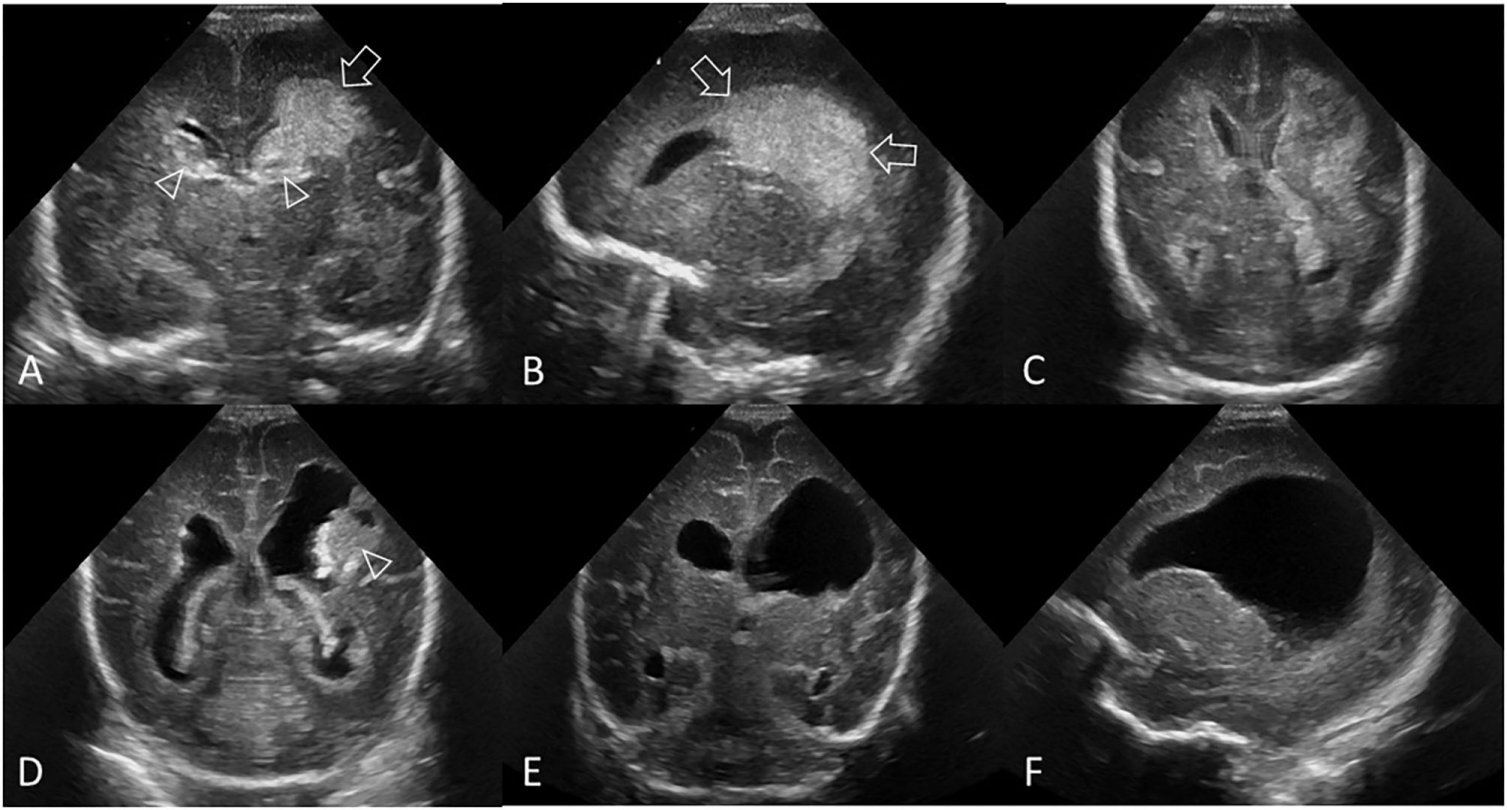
Grade III GMH-IVH + PVHI. Ultrasound scan in a preterm infant (GA 24 weeks) with bilateral GMH-IVH and left-sided PVHI. (A) Coronal scan 2 days after birth showing a left-sided grade III GMH-IVH (arrowhead, AHW = 7 mm, measurement not shown) and large echodensity in the left frontoparietal white matter (arrow). Smaller right-sided grade II GMH-IVH (arrowhead, AHW = 4 mm, measurement not shown). (B) Left parasagittal scan showing the periventricular echodensity (arrows) extending from the posterior frontal white matter to the parietal white matter. (C) Follow-up coronal scan 2 weeks after birth shows the area of PVHI beginning to develop central echolucency, indicating early cystic degeneration. (D) Follow-up coronal scan 7 weeks after birth shows further cystic degeneration following the PVHI with echogenic clot debris, dilated lateral ventricles, and echogenic ependymal lining. (E,F) Follow-up coronal and left parasagittal scans at 12 weeks show the large porencephalic cyst communicating with the lateral ventricle. Internal clot debris has cleared and ependyma is no longer echogenic. The persisting ventriculomegaly is now partially passive (i.e., ex-vacuo) in origin. In this example, the independent recording of the bilateral GMH-IVH patterns on the baseline cUS exam is recommended.
